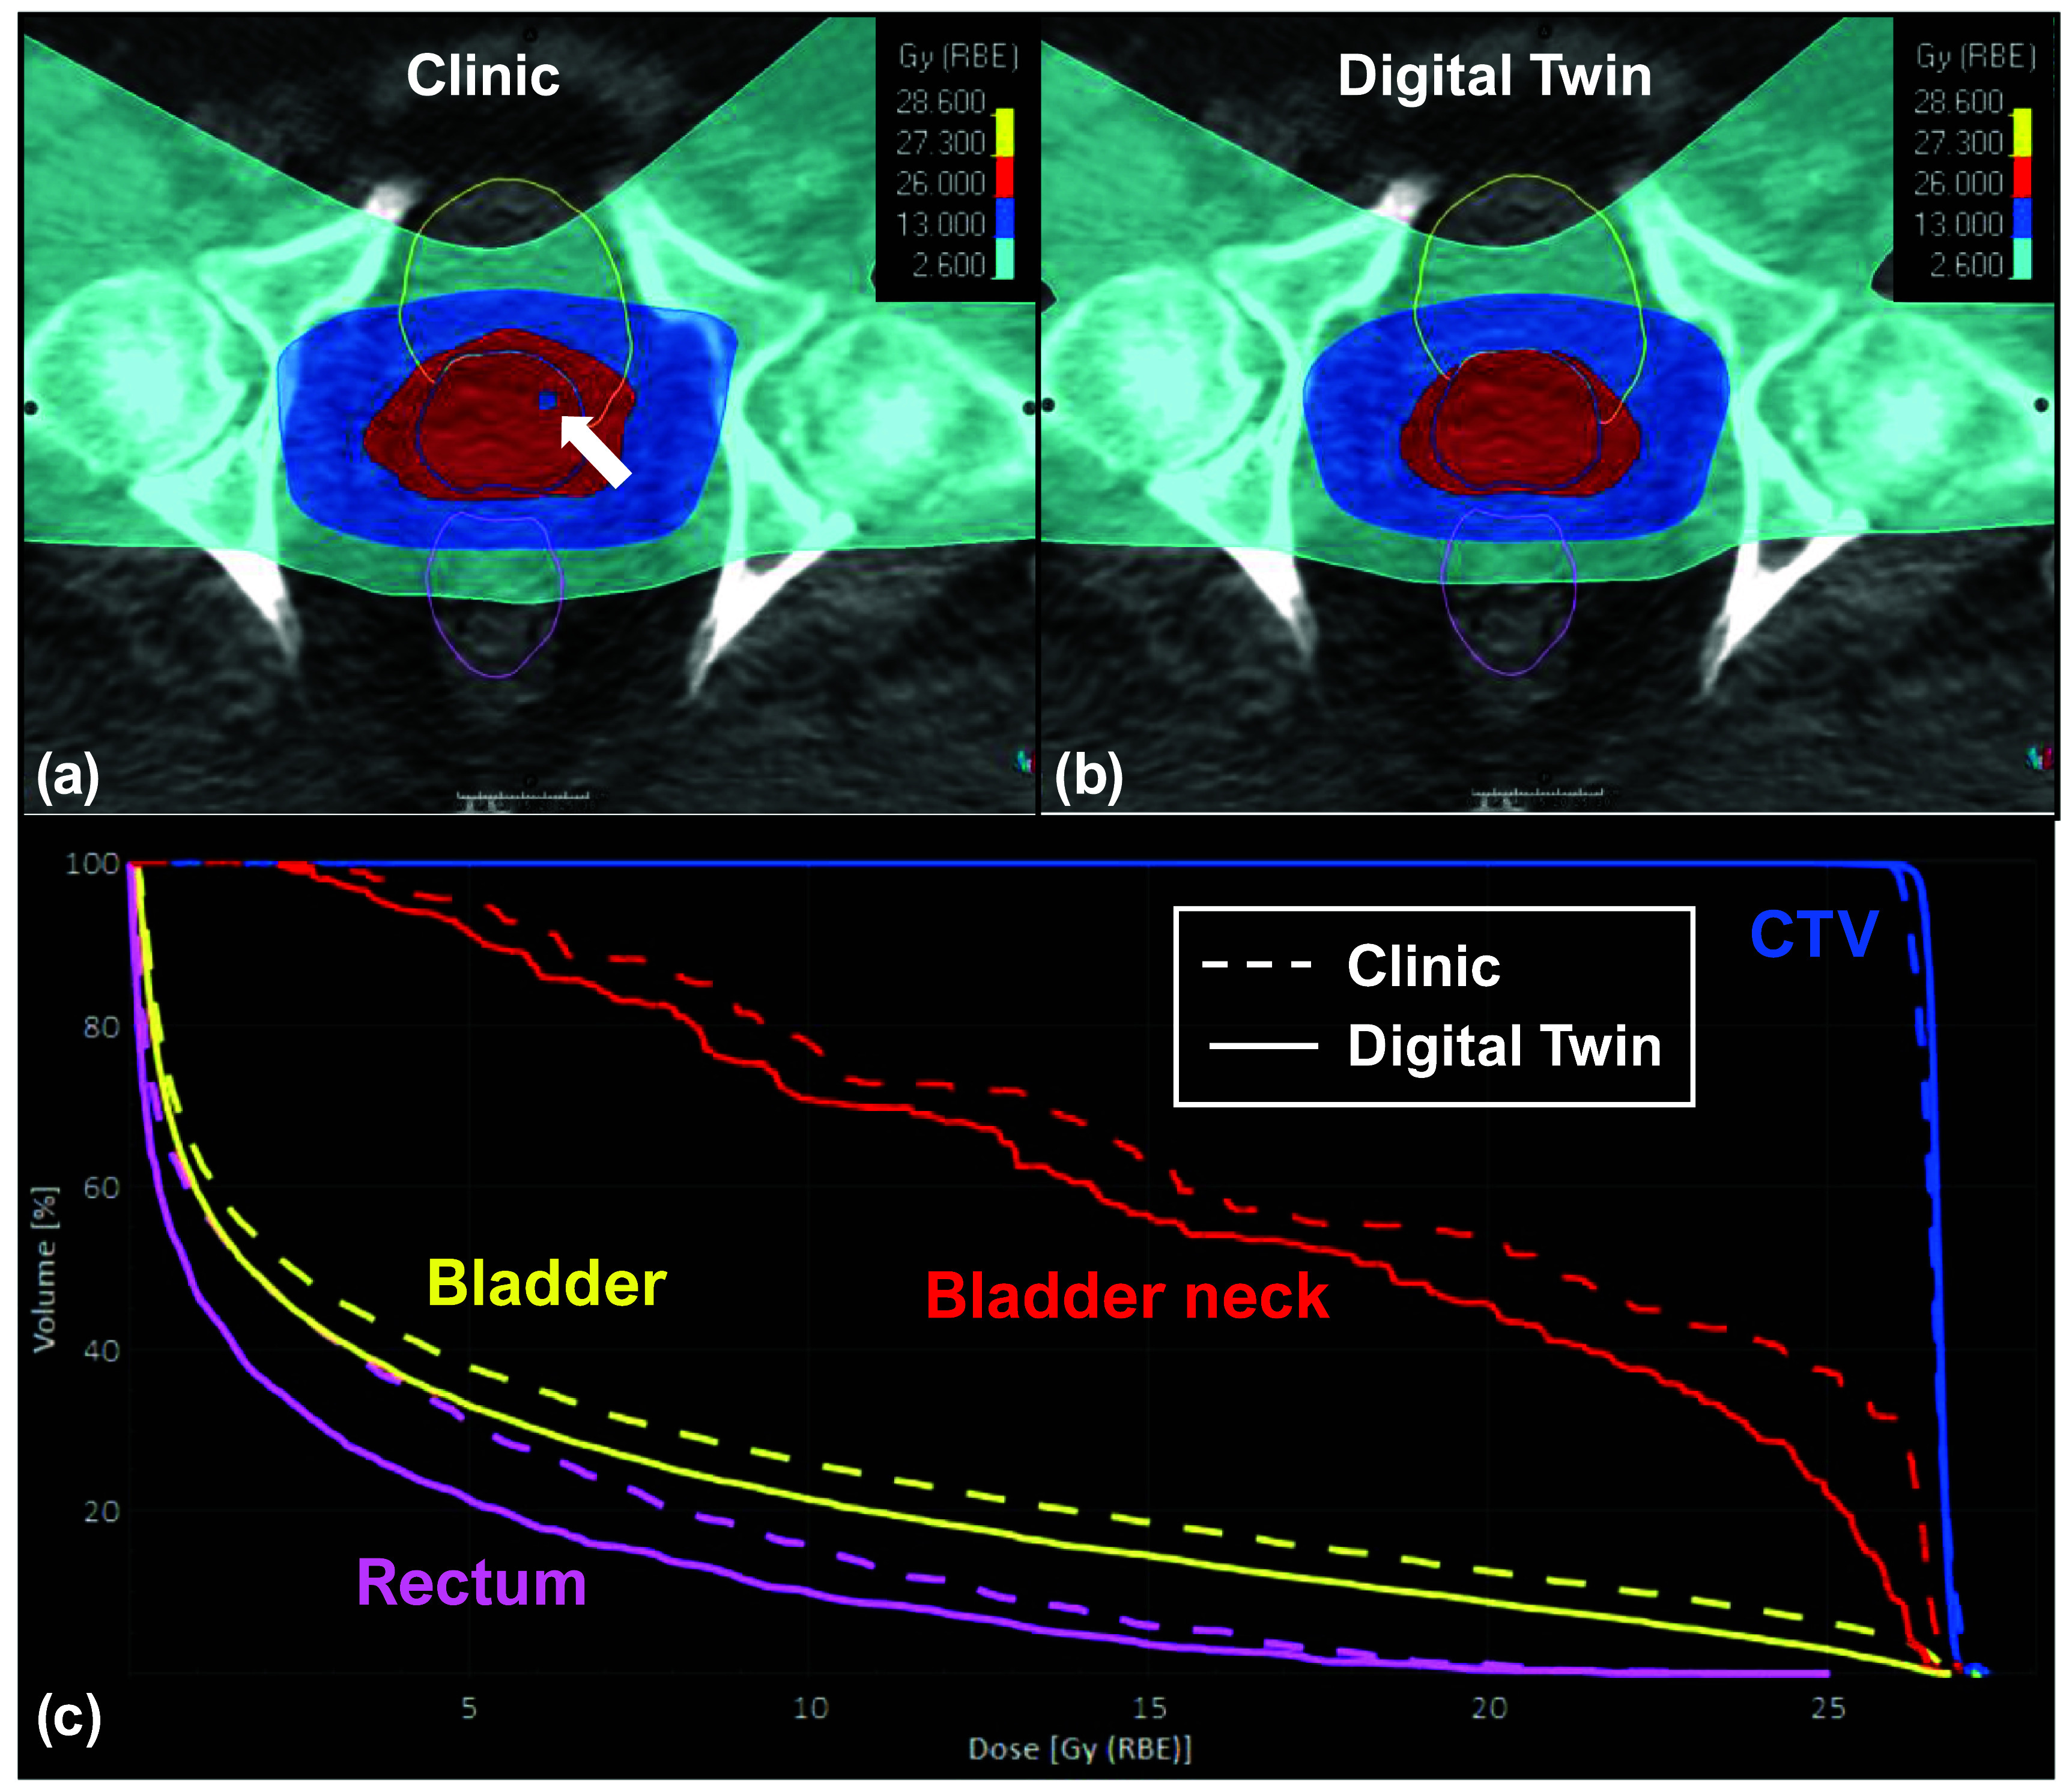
Dosimetry comparisons for Patient 7 between the clinical and DT-based plans. The top row displays the dose distributions in color wash for the (a) clinical plan and (b) DT-based plan, overlaid with transversal CT images and the contours of CTV (blue lines), bladder (yellow lines), and rectum (magenta lines). The bottom row (c) displays the dose-volume histogram (DVH) for CTV and OAR structures, where the dashed and solid lines represent the clinical plan and DT-based plan. The white arrow indicates the cold spot location in CTV.
